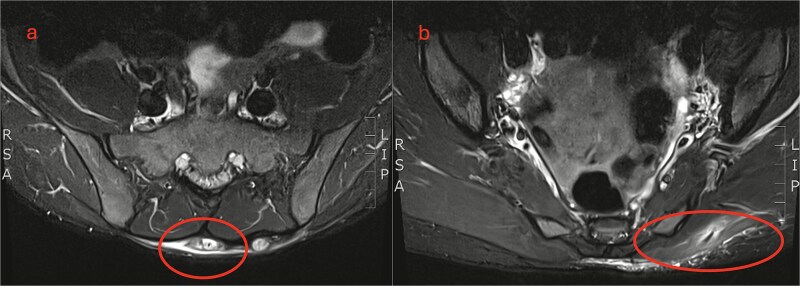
MRI lumbosacral spine. (a) Axial view shows a soft tissue mass overlying the sacrum. (b) Sagittal view shows a linear extension tracking down to the left gluteus maximus.
